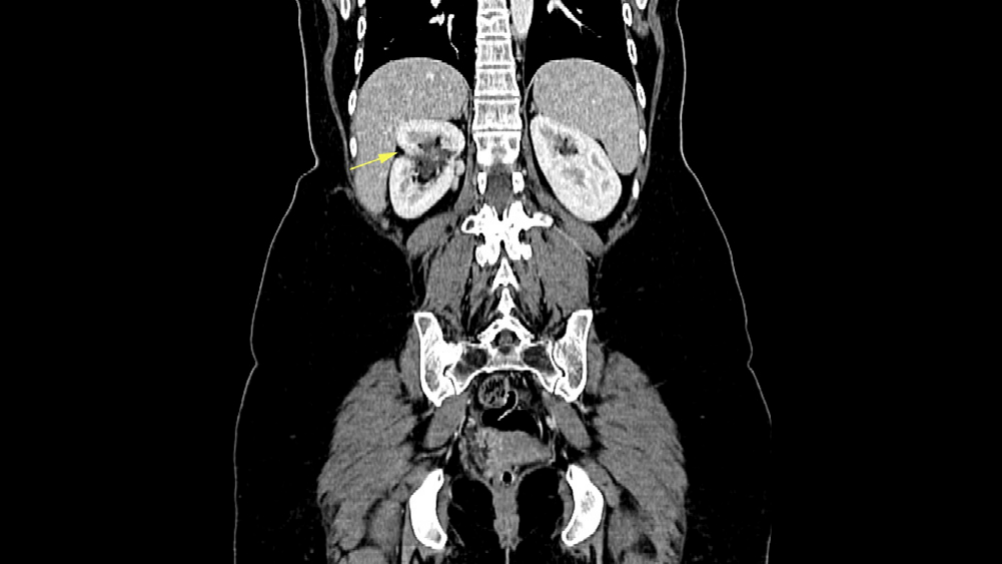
Coronal reformatted contrast-enhanced abdominal CT shows signs of operation at the mid portion in the form of renal cortical discontinuity (arrow).
